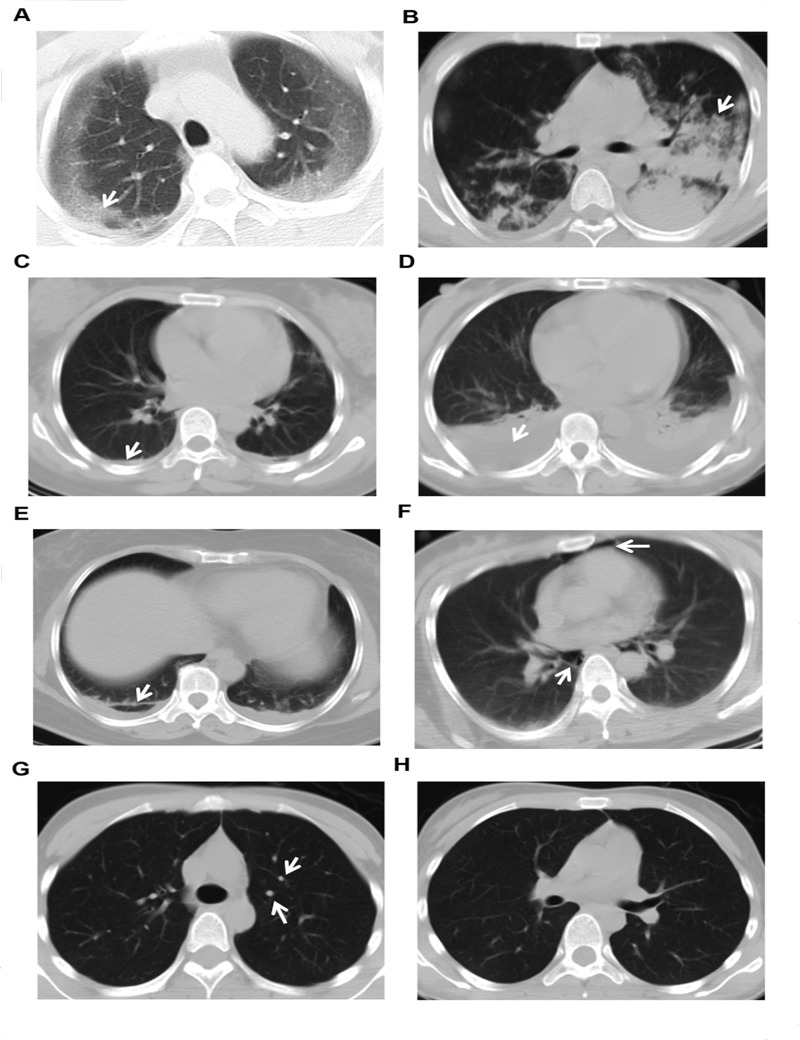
Eight CT findings of PQ poisoning captured within the first 5-day. A. ground glass opacity (GGO); B. consolidation; C. pleural thickening; D. hydrothorax; E. fibrosis; F. pneumomediastinum; G. nodule; H. “no obvious lesion”. Arrows represented the lesions.
